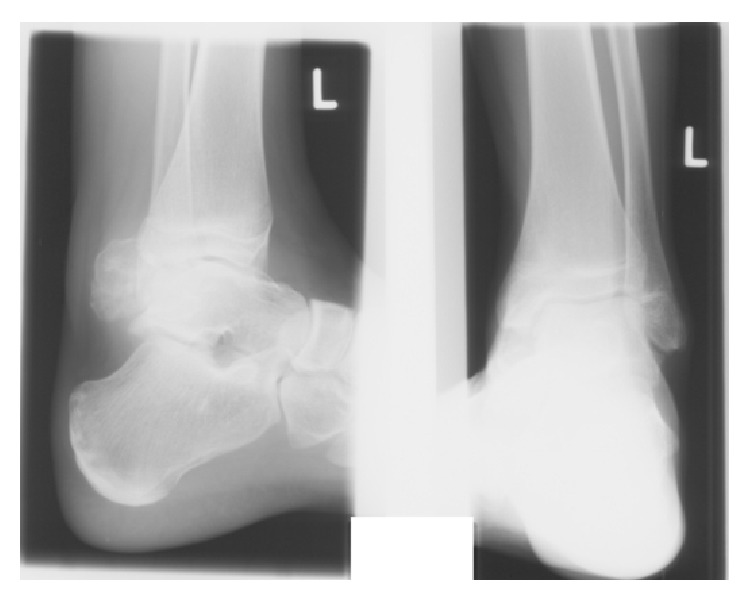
Plain radiography, anteroposterior and mediolateral view of the ankle joint.
